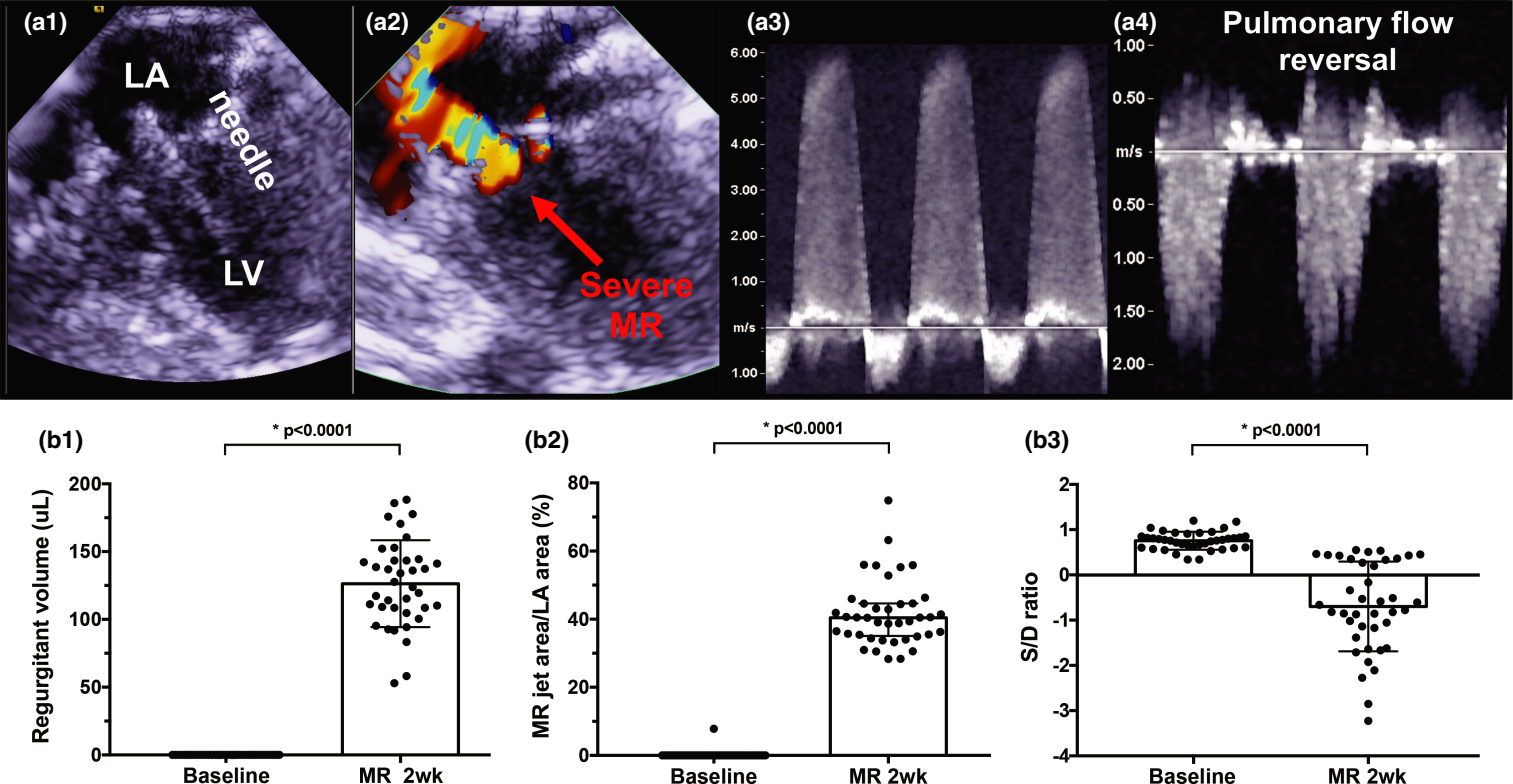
Creation and validation of volume overload induced severe mitral regurgitation. (a1) Two‐chamber transesophageal echocardiographic view visualizing the 23G needle perforating the mitral leaflet; (a2) color Doppler image indicated the MR jet; (a3) MR jet visualized on a pulsed‐wave Doppler image; (a4) severe MR resulting in pulmonary flow reversal; (b1) regurgitant volume; (b2) MR jet area normalized to left atrial area; (b3) pulmonary venous flow systolic to diastolic (S/D) ratio. *p* < 0.05 was considered significant. MR, mitral regurgitation.
